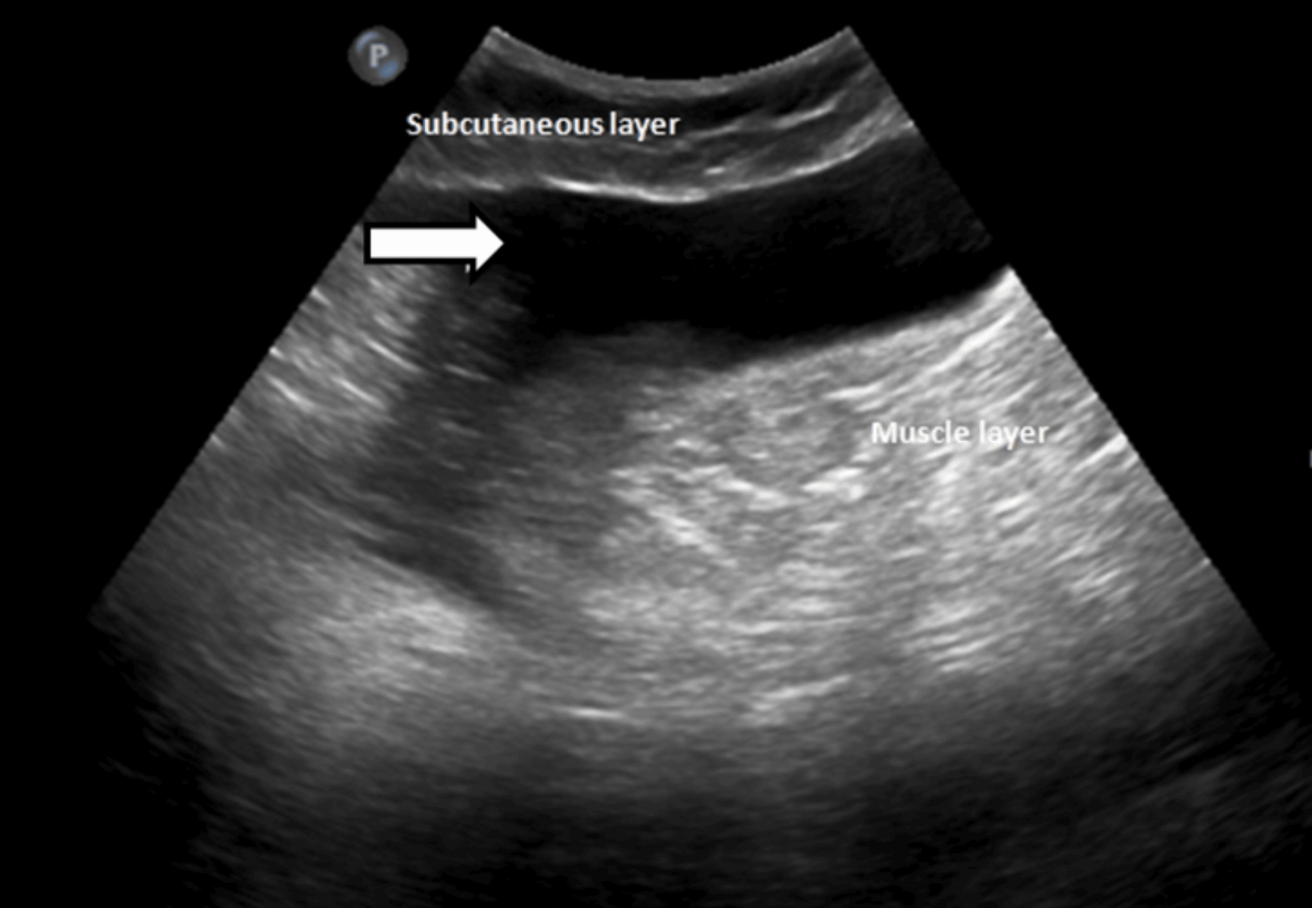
Ultrasound image showing an anechoic fluid collection of volume ~80-90cc noted between the subcutaneous layer and muscle (white arrow) of the right thigh (region of interest).
